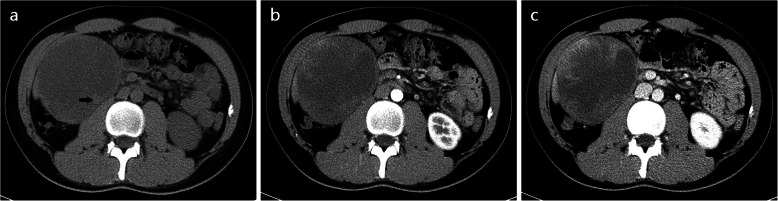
Hepatic undifferentiated embryonal sarcoma in a 22-year old man. Axial CT image shows a protruding bulging mass in hepatic segment VII with a large cyst-like area (a). Contrast-enhanced dynamic CT axial images present the mass hypo-enhanced peripherally on the AP (b) and centripetally in the PVP (c)
